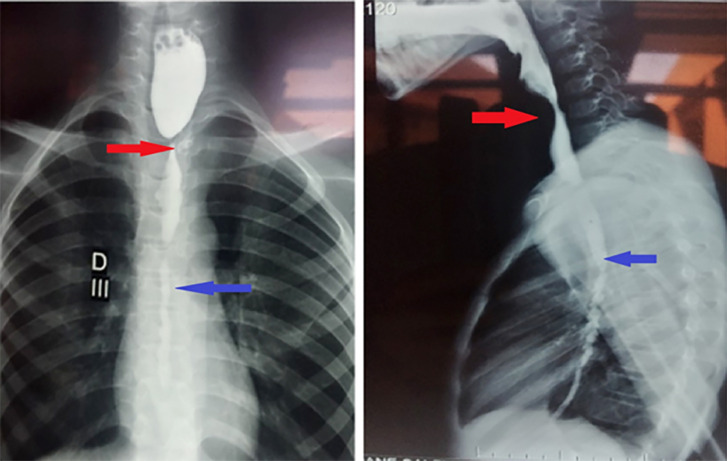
Cliché de TOGD face et profil, montrant une sténose de l’œsophage cervical (flèches rouges), associée à un long rétrécissement de l’œsophage thoracique (flèches bleues) Face and profile esophagogastroduodenal transit radiograph, showing stenosis of the cervical oesophagus (red arrows), associated with a long narrowing of the thoracic oesophagus (blue arrows)
